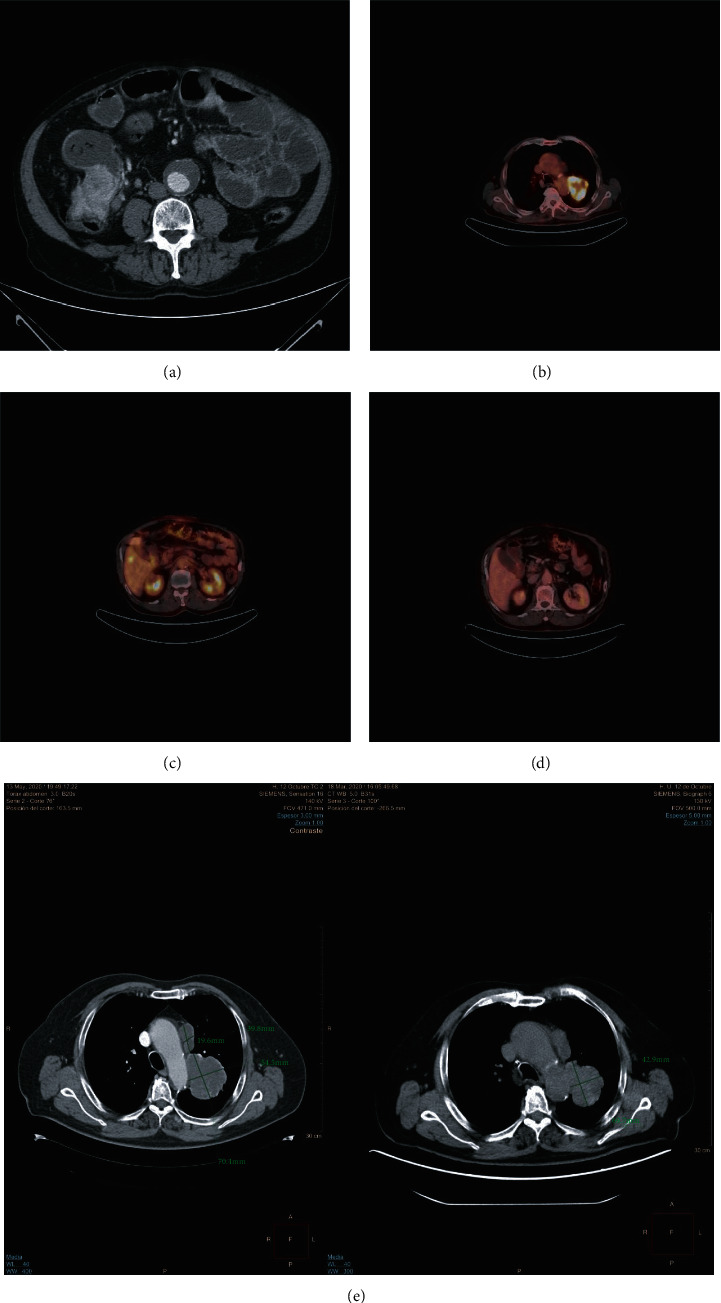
(a) Initial diagnostic CT scan shows an ileocecal mass of approx. 7 cm of diameter, with no clear radiologic signs of bowel distention or obstruction. (b) Initial PET/CT scan shows a 7 cm FDG-avid paramediastinal lesion in stretch contact with the aortic arch. (c) Initial PET/CT scan shows a metastatic lesion at the hepatic segment V. (d) Control PET/CT scan after 2 cycles of carboplatin/etoposide shows complete resolution of the hepatic lesions. (e) Control CT scan after 2 cycles of carboplatin/etoposide and 3 cycles of carboplatin/paclitaxel shows tumor progression (left image) of the paramediastinal mass, further compromising the aortic arch.
